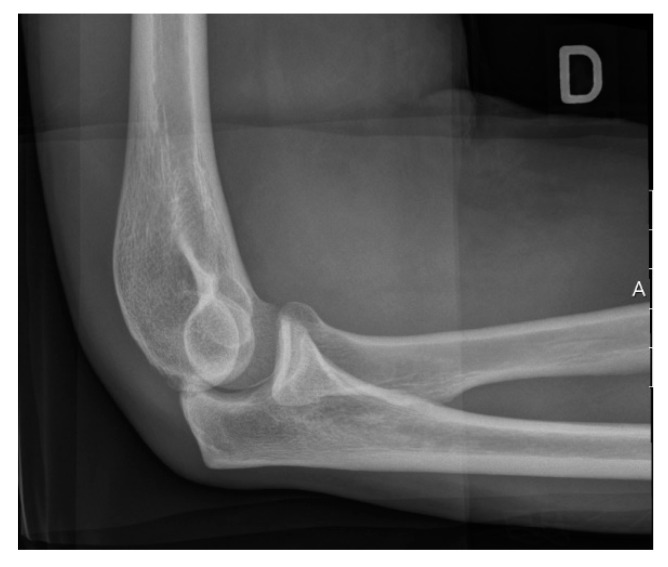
Lateral radiograph of the elbow in a healthy patient.
